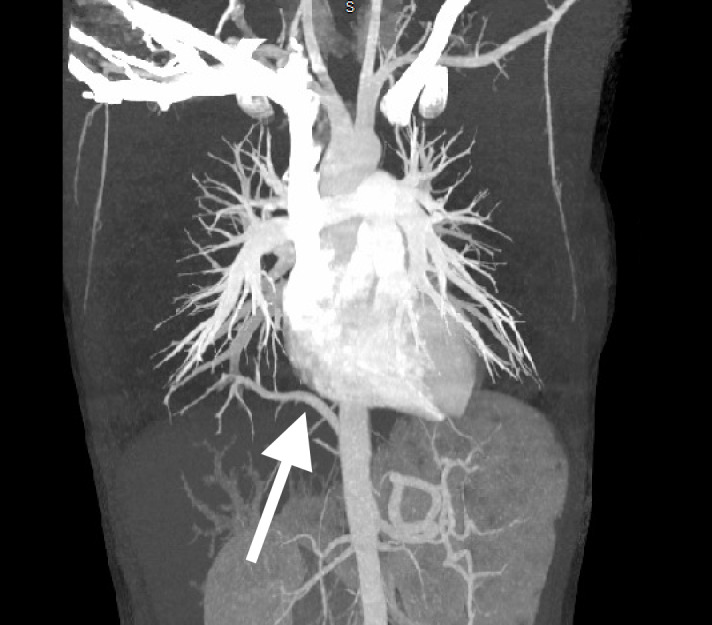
Computed tomography angiogram demonstrating the right lower lobe pulmonary artery arising from the descending aorta beyond the level of the diaphragm (arrow).
